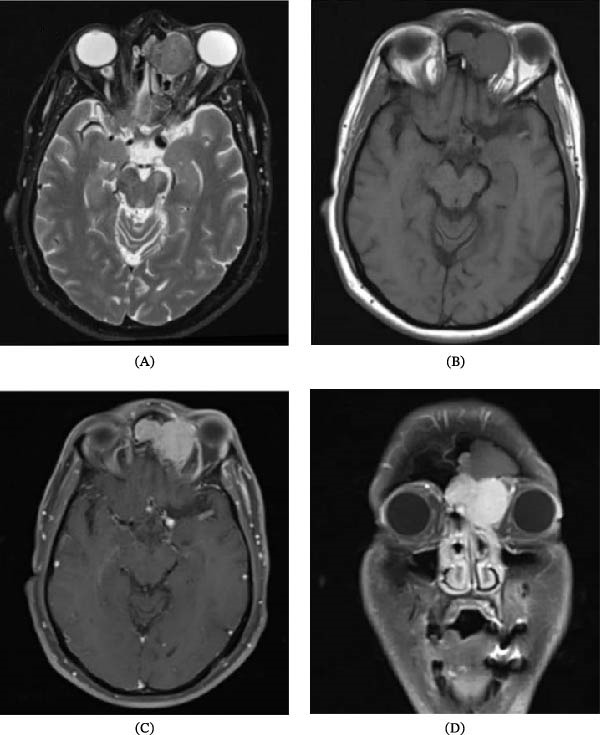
Multiplanar brain MRI with gadolinium images: (A) axial T2, (B) axial T2, (C) axial T1 with contrast, and (D) coronal T1 with contrast: the mentioned mass at the left anterior ethmoid air cells showing a solid signal intensity which is isointense to gray matter on both T1 and T2, with avid homogenous contrast enhancement.
